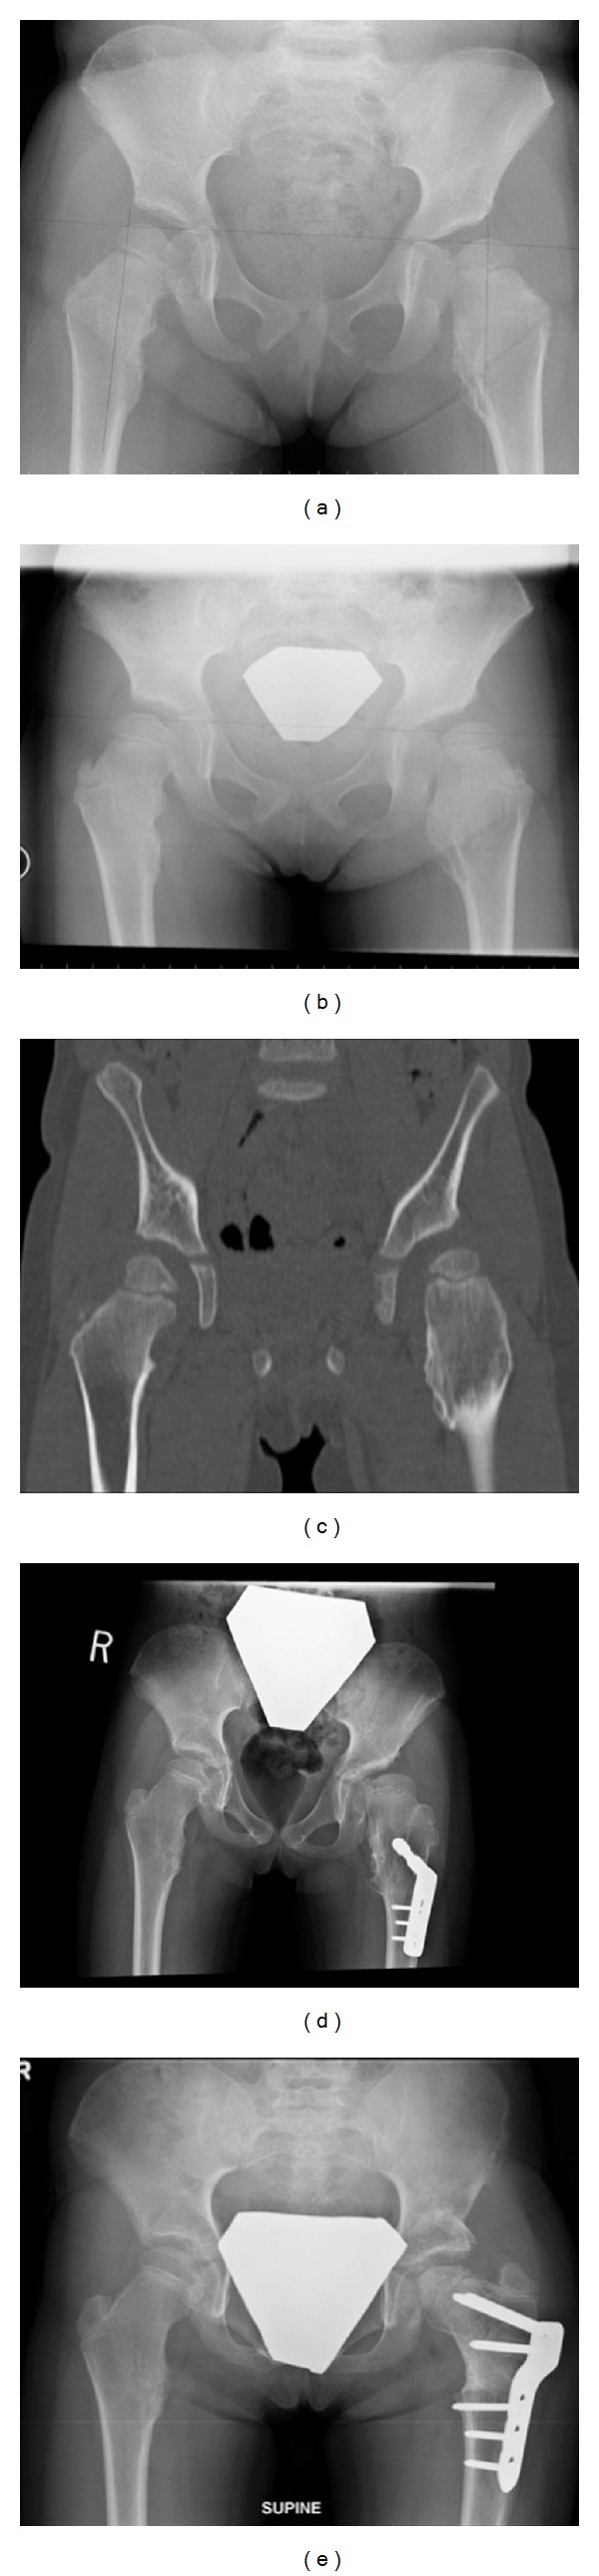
(a) Anteroposterior pelvic radiograph at initial presentation. (b) Anteroposterior pelvic radiograph and (c) Computed tomography of the pelvis at one-year follow-up. (d) Anteroposterior pelvic radiograph 4 years after left femoral varus derotational osteotomy (VDRO) with partial excision of the osteochondroma. (e) Anteroposterior pelvic radiograph ten months after performing the second VDRO, modified Dega osteotomy, and extensive excision of femoral neck osteochondroma. Partial left femoral head necrosis is also noted.
